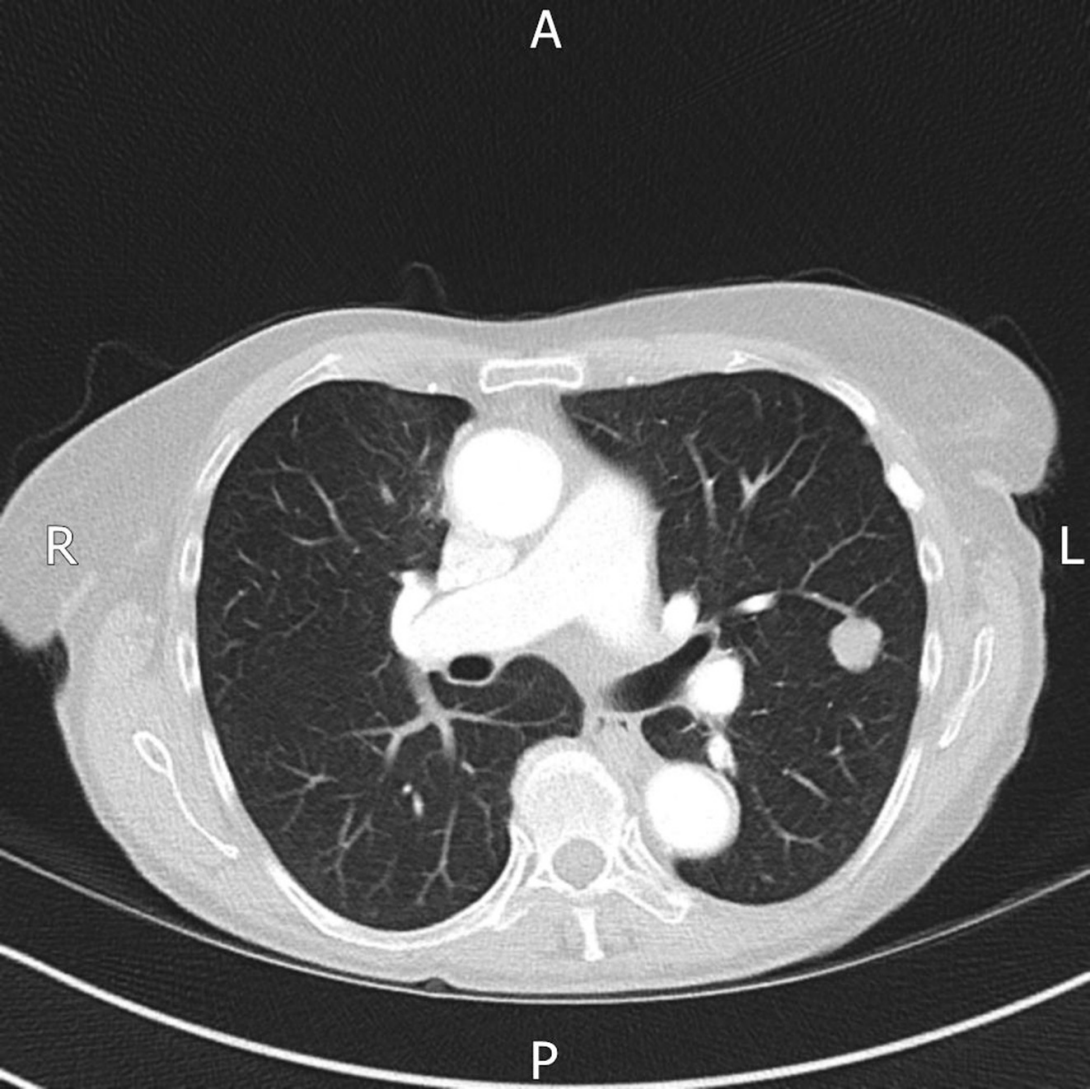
CT scan of thorax shows 1.2 cm pulmonary nodule. No associated mediastinal lymphadenopathy is seen. No other discrete nodules are present.
